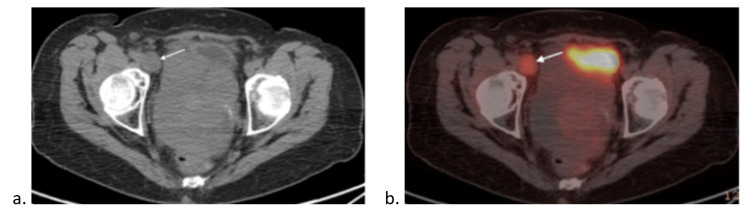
(a) PET scan and (b) CT scan demonstrating a hypermetabolic right external iliac node (white arrows)
